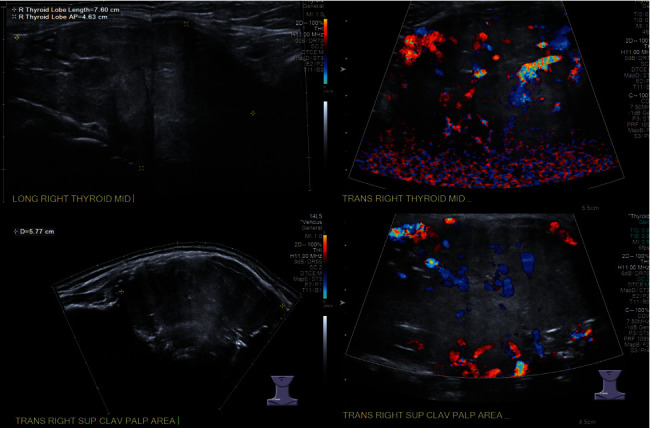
Ultrasound image demonstrating a diffuse mildly heterogeneous enlargement of the right thyroid lobe (a). The associated increased flow within the right thyroid lobe on the color Doppler image (b). A soft tissue mass also seen in the region of palpable concern in the right supraclavicular area, measuring 5.77 cm (c). The associated internal flow in the mass on the color Doppler image (d).
